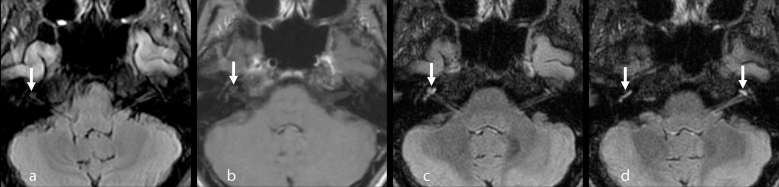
Inner ear findings on MRI. A faintly increased signal is suspected in the right cochlea on two-dimensional FLAIR image (arrow in a). There are no abnormal findings in the left cochlea. Gd-enhanced 3D *T*_1_ weighted image shows a faint enhancement in the right middle turn of the cochlea (arrow in b). Gd-enhanced 3D FLAIR images shows more conspicuous enhancement in the right apical to basal turn of the cochlea (arrow in c and right arrow in d) and left apical turn of the cochlea (left arrow in d) compared with Gd-enhanced 3D *T*_1_ weighted image (3D FLAIR images are multiplanar reconstruction images, with the location and slice thickness adjusted to two-dimensional FLAIR). FLAIR, fluid attenuation inversion recovery; 3D, three-dimensional.
